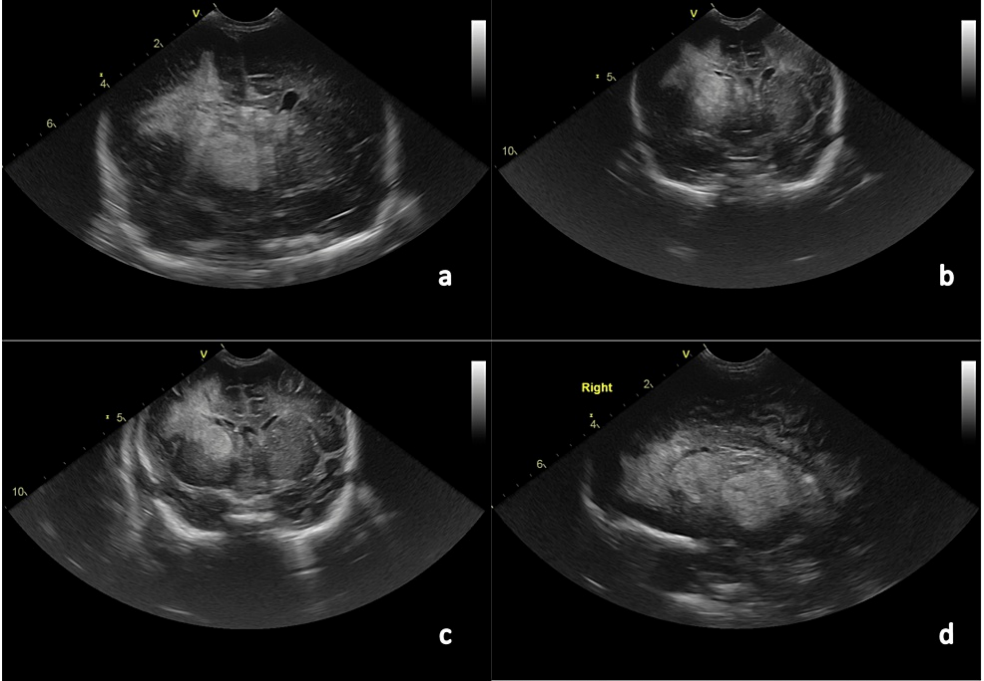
Cranial ultrasound showing tetraventricular hemorrhage with extensive right parenchymal hemorrhage, midline shift, and severe left fronto-parieto-occipital hemorrhage A,B,C: coronal views. D: parasagittal view.
